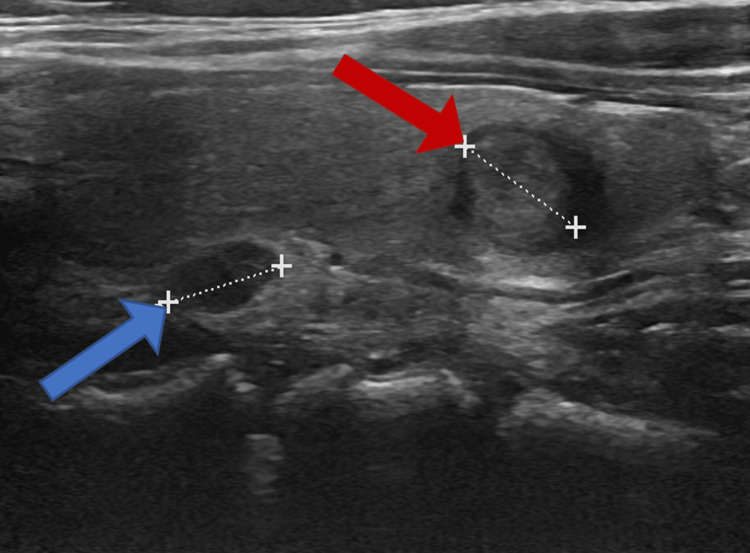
Left thyroid ultrasound showing a 0.8cm posterior thyroid nodule suggestive of parathyroid adenoma (blue arrow) and one of the many unchanged thyroid nodules, 0.9cm in size (red arrow)
